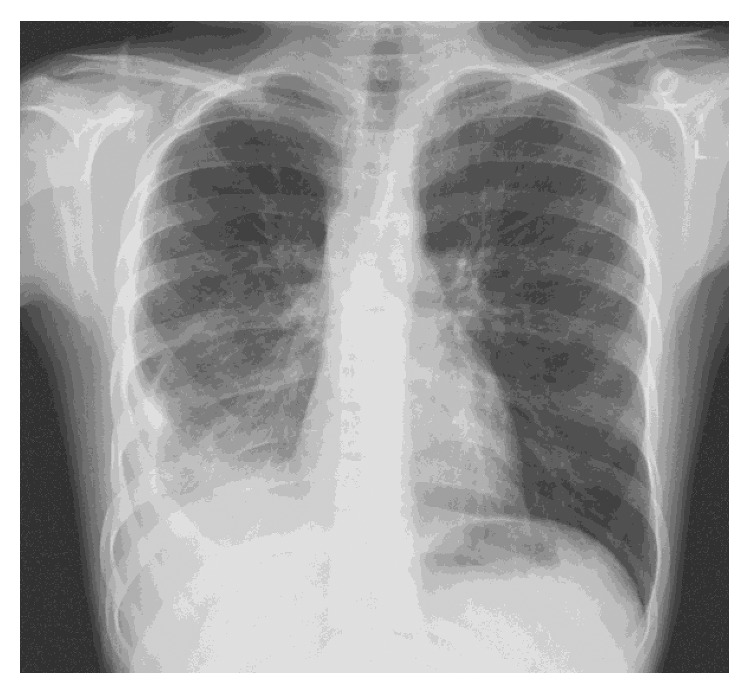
Posteroanterior chest radiography at presentation showing right lower lobe consolidation with pleural thickening and effusion, resulting in marked right lower lobe volume loss.
